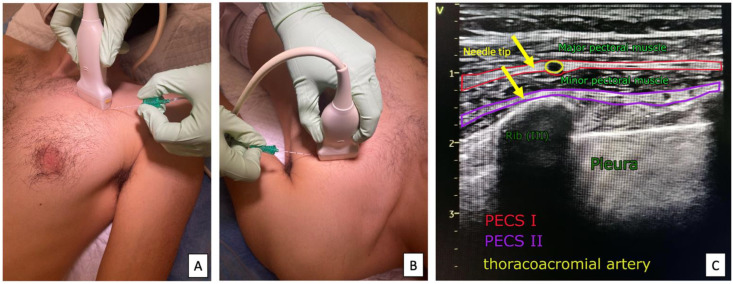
Ultrasound transducer positioning of the PECS I (A) and PECS II (B) blocks and sonoanatomy of the PECS blocks (C).
